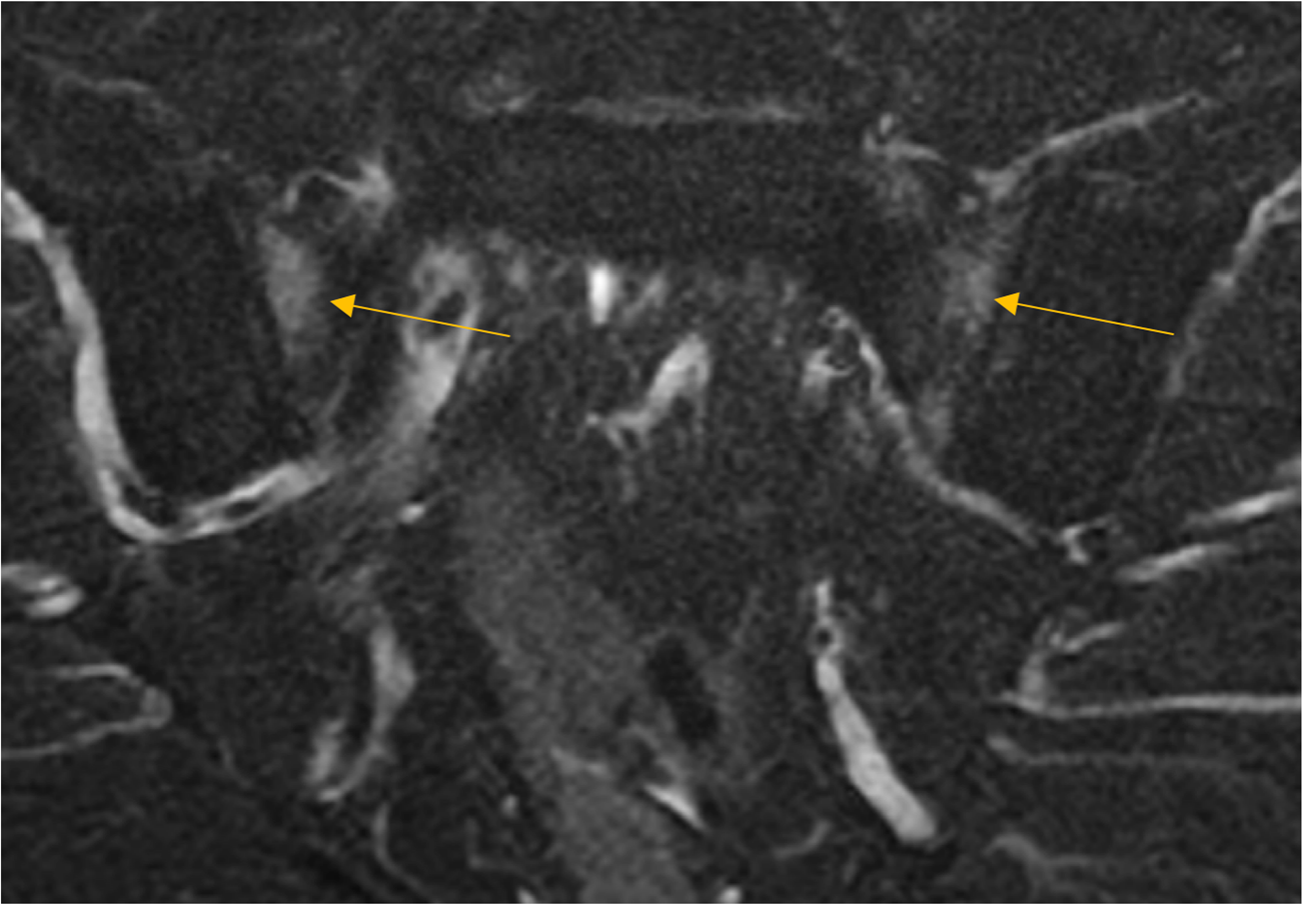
Magnetic resonance imaging (MRI) of the sacroiliac joints (SIJ) showing bilateral sacral joint bone marrow edema on in a patient with early psoriatic arthritis. The Bone marrow edema is more florid at the anterior part of the joint adjacent to the capsular enthesis. It remains to be determined whether the patterns of inflammation at entheses in soft tissue or in the bone may influence responses to therapy
